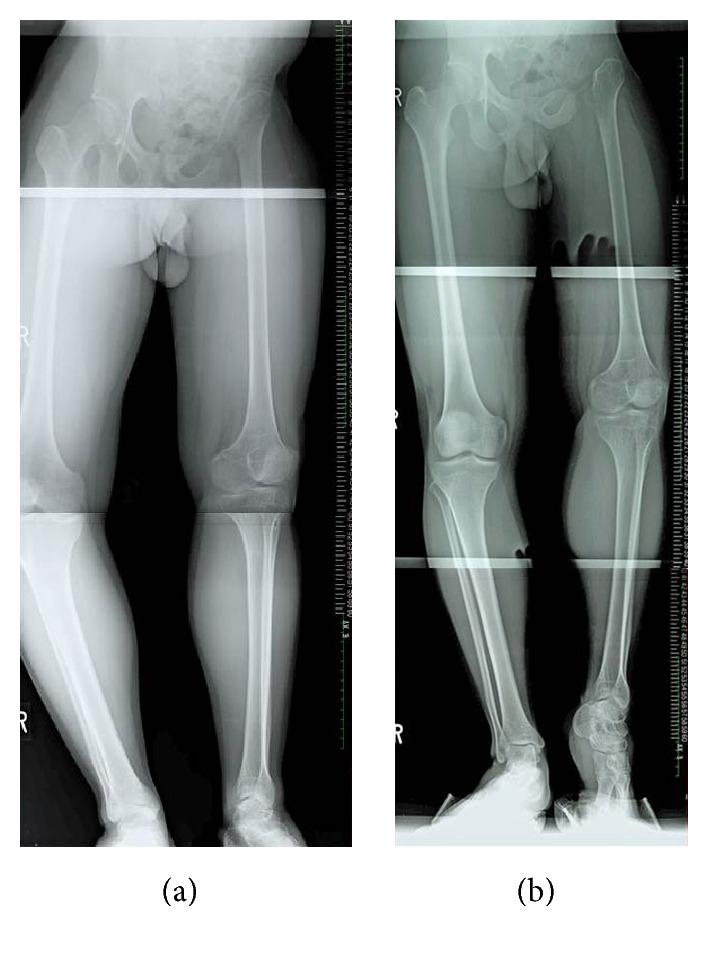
Radiographs of entire lower limbs before leg lengthening showed tip of the residual left femur had moved upward about 4 cm in standing position (a) compared with the right leg (b).
